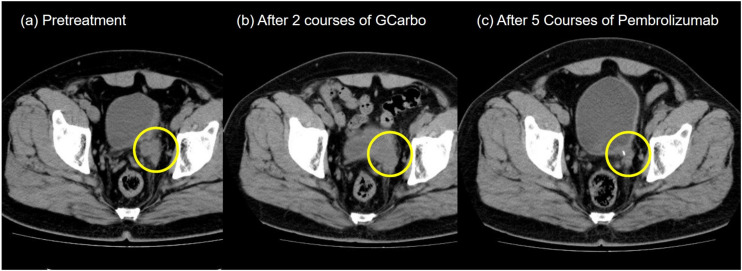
Pelvic computed tomography during treatment. (a) Pretreatment. A left ureteral tumor was observed. (b) After two courses of gemcitabine plus carboplatin chemotherapy. (c) After five courses of pembrolizumab.
